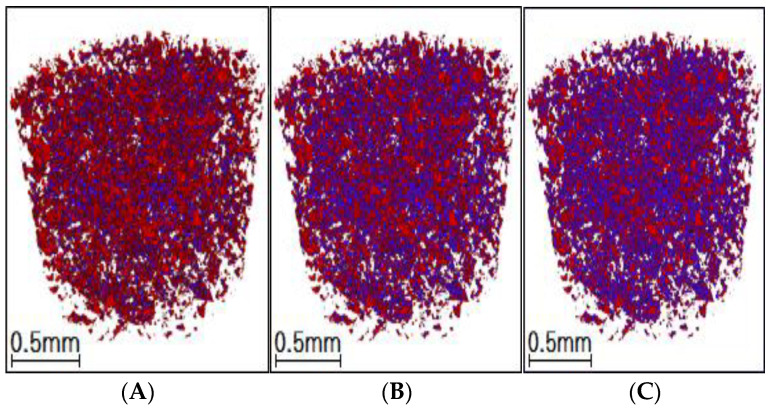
Distribution map generated from 3D CT scans of remaining oil. (A) Initial; (B) water flooding; (C) heavy oil activator flooding.
